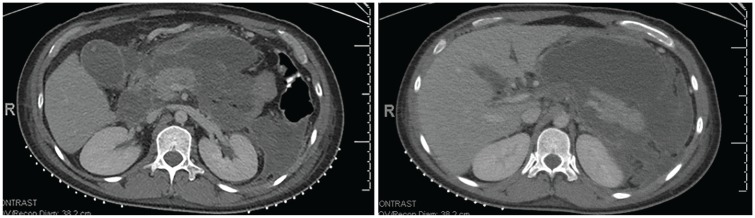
Computed tomography scan notable for a large peripancreatic fluid collection most consistent with walled-off necrosis.
